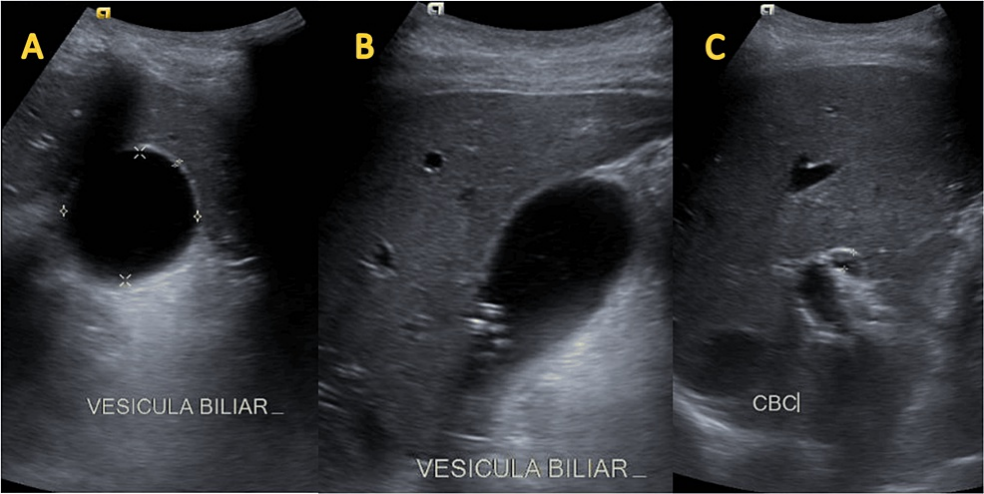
Ultrasound of the upper abdomen shows a gallbladder with 2 mm walls (A) containing multiple stones (B) and a 6 mm common bile duct (C)
